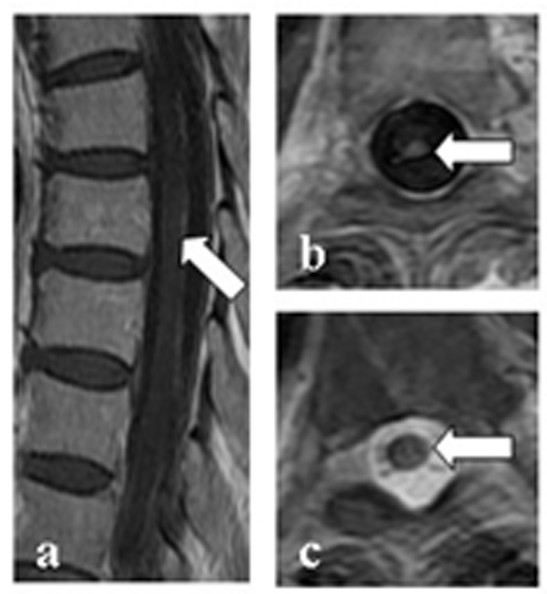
Spinal MRI of a CNS-TB patient with spinal cord involvement. a–b: meningeal and whole lesion enhancement on a T1-weighted contrast image; c: T2-weighted image showing an isolated lesion at the T5 level of the spinal cord.
